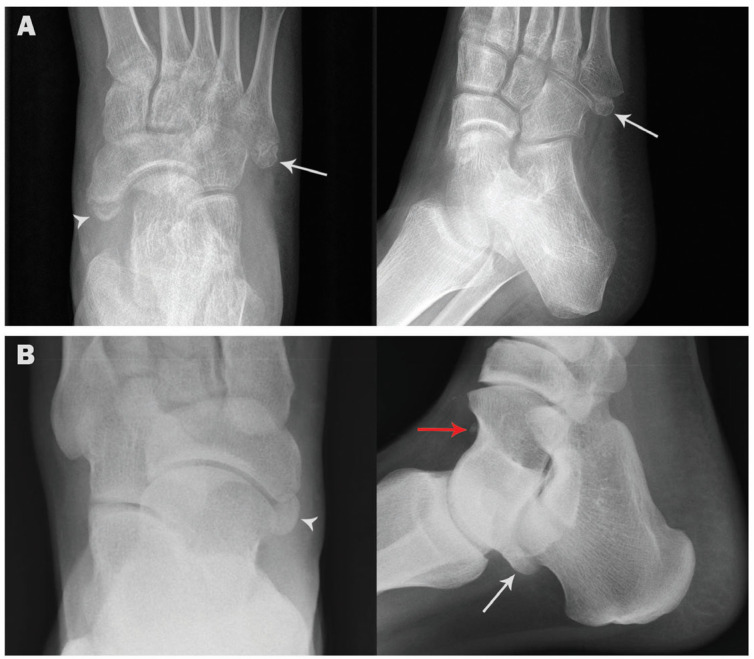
Coexistence of accessory navicular and other ossicles in the same foot. (A). AP and oblique radiographs showing accessory navicular (arrowhead) and os vesalianum (arrow) in a 56-year-old female. (B). AP and lateral radiographs showing accessory navicular (arrowhead), os trigonum (white arrow) and os supratalare (red arrow) in a 45-year-old male.
